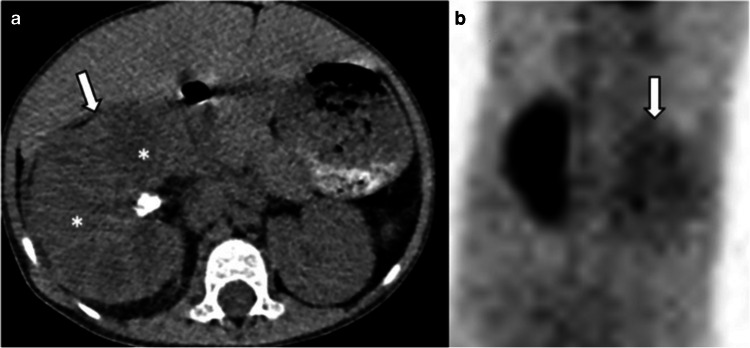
Xanthogranulomatous pyelonephritis. A 7-year-old girl was admitted to the hospital with right flank pain and vomiting. a Axial non-contrast computed tomography image shows a right pelvic stone, enlarged right kidney (*arrow*), and bear’s paw sign (*asterisk*). b Scintigraphy with 99mTc-diethylene-triamine-pentaacetate demonstrates severely decreased function of the right kidney, with a relative contribution of only 10% (*arrow*)
